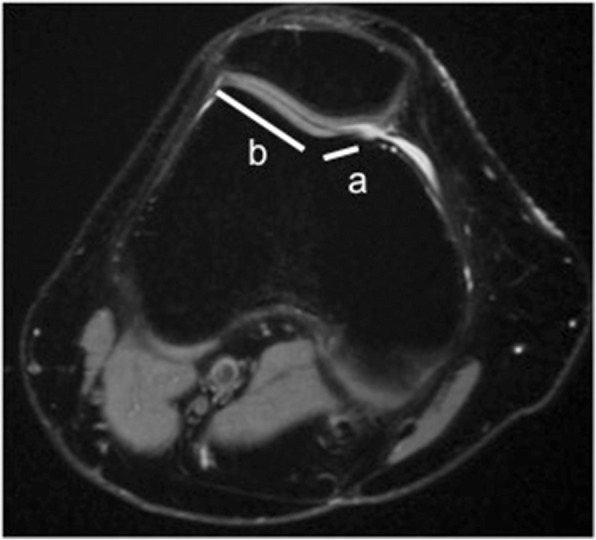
Facet asymmetry assessment for trochlear dysplasia on axial MRI. It is the percentage of the medial (a) to the lateral (b) trochlear facet length (a/b × 100%)
